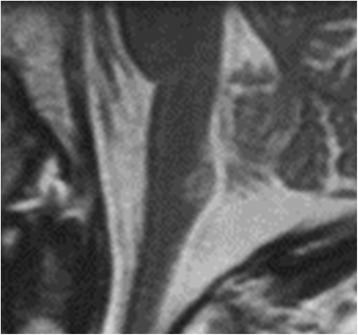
Sagittal T2-weighted imaging revealing a lesion involving the caudal medulla
